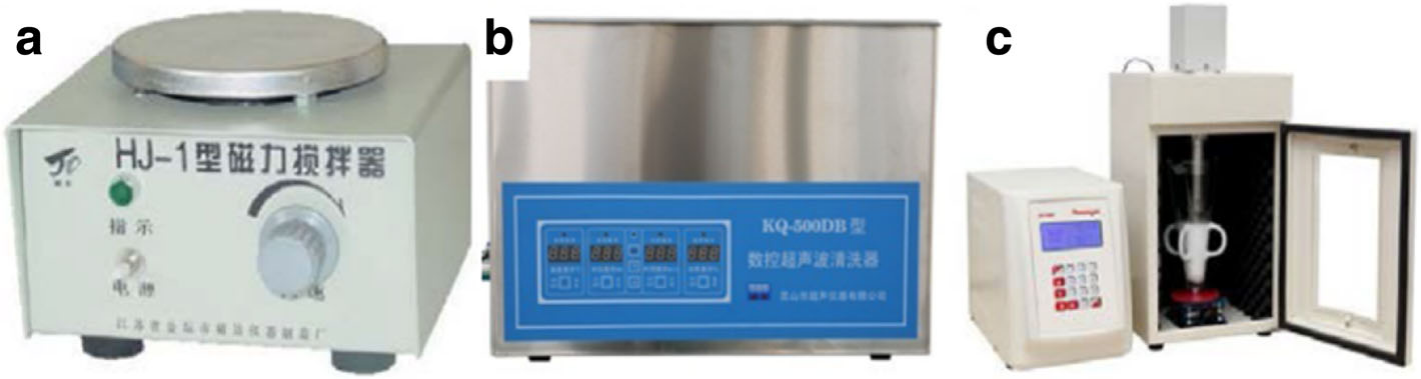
Images of dispersion instruments. a Magnetic stirrer. b Ultrasonic bath. c Probe
